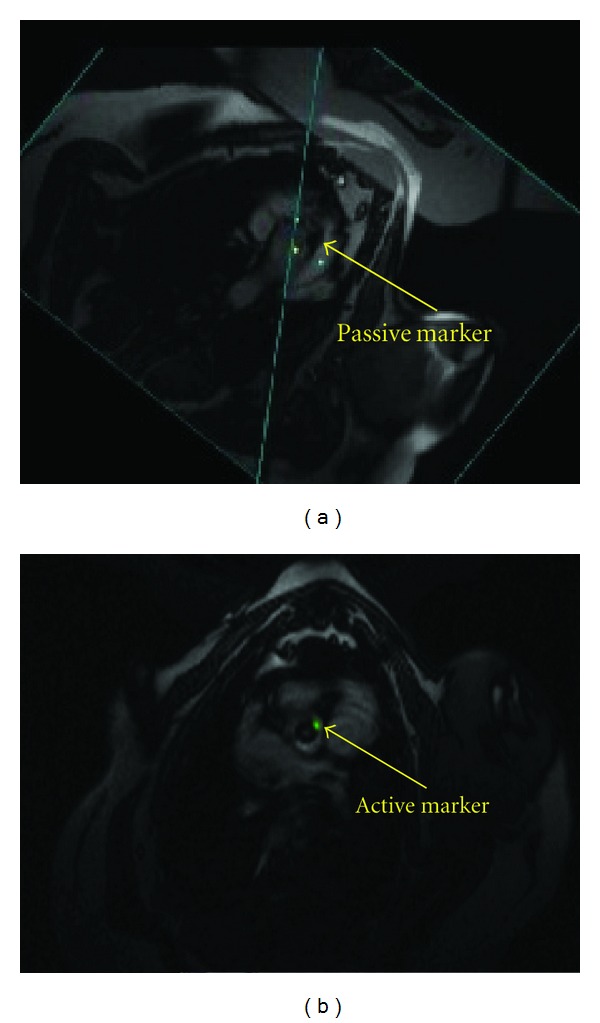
(a) Passive marker showing black signal in MRI. (b) Active marker showing bright signal and highlighted in green. These markers are used to indicate the orientation of the prosthesis in an MRI-guided aortic valve implantation procedure.
